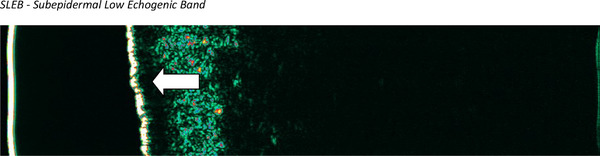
High‐frequency ultrasound image in active subacute cutaneous lupus erythematosus (facial skin). The arrow indicates SLEB. SLEB, subepidermal low echogenic band.
